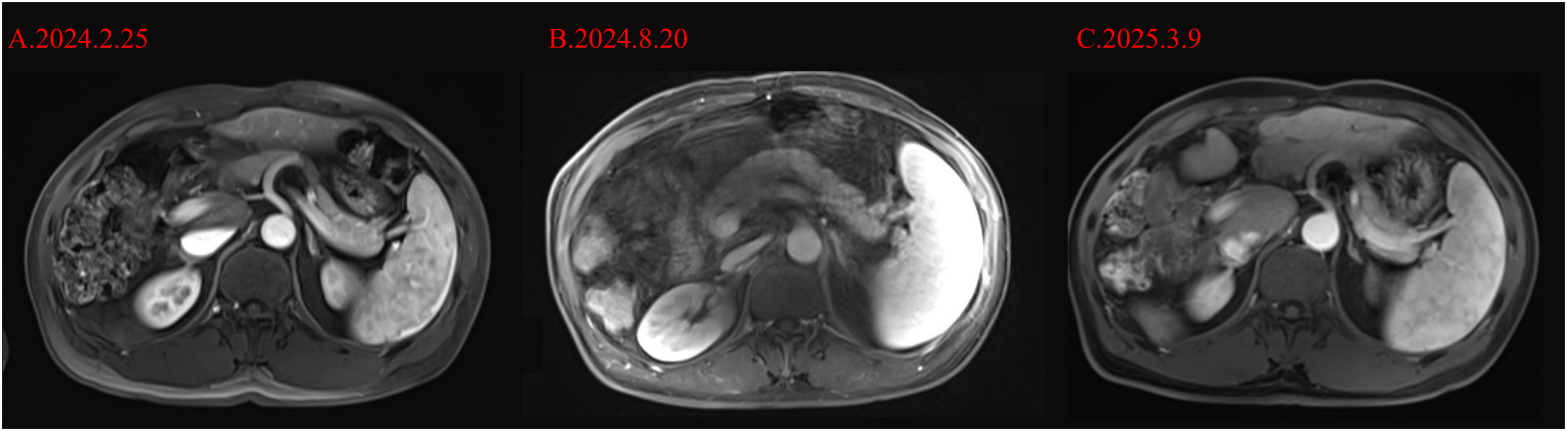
MRI changes of the adrenal glands before and after the occurrence of adrenal crisis in the patient. (A) shows the MRI image of the adrenal glands of the patient before the occurrence of adrenal crisis (2024.2.25), (B) shows the MRI image of the pituitary gland of the patient on the third day after the occurrence of adrenal crisis (2024.8.20), and (C) shows the MRI image of the adrenal glands of the patient about half a year after the occurrence of adrenal crisis.
